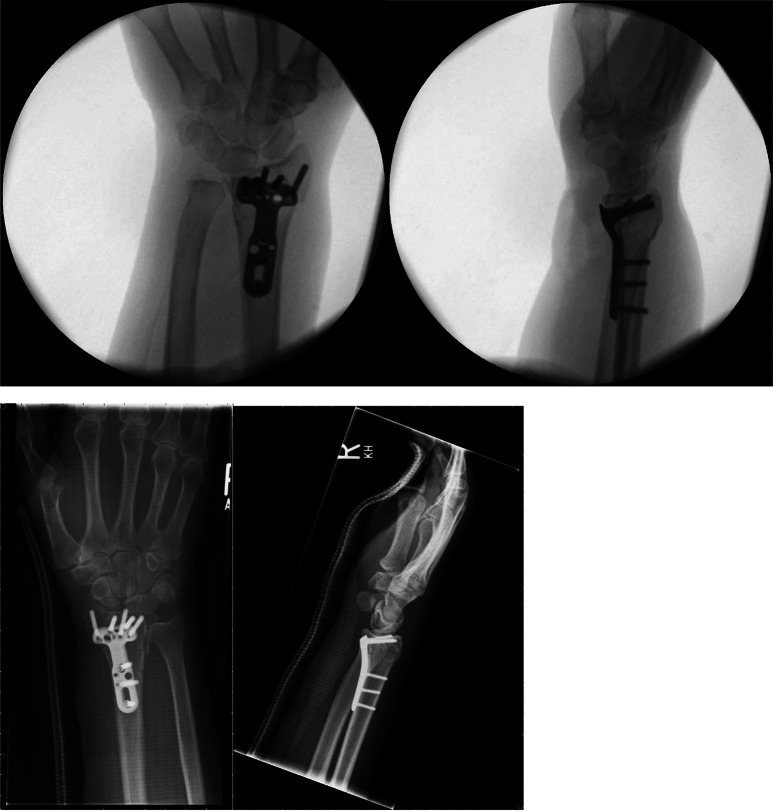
Patient 2: intraoperative imaging without abormalities, postoperative radiographs with suspected intraarticular screw positioning
